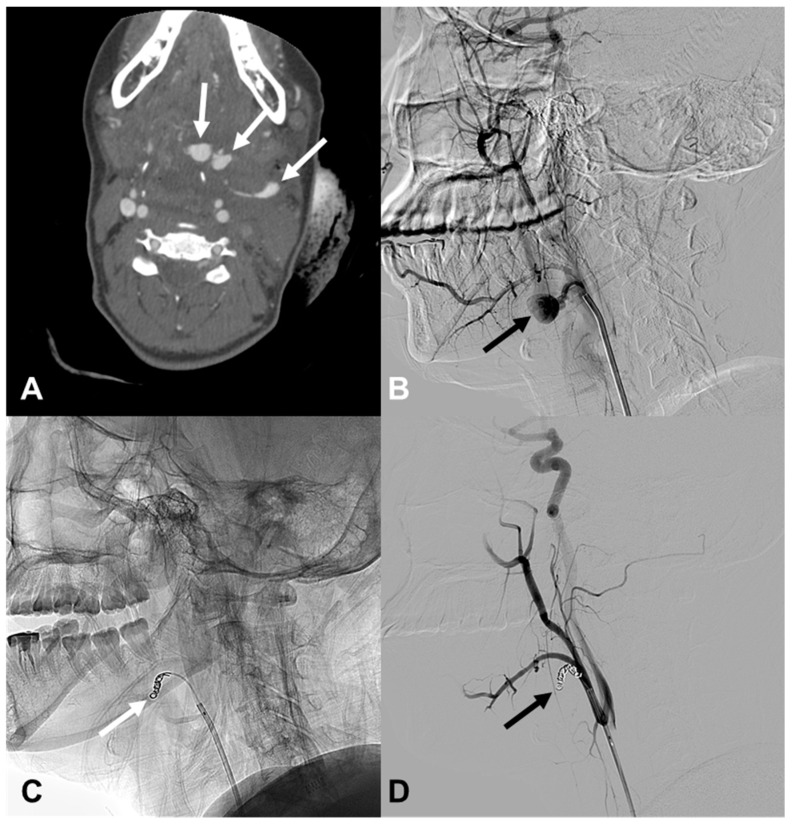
A 22-year-old woman (patient 14) with a stab injury in the left lingual artery. (A) Axial CT shows contrast extravasation (arrows) in the left level I and II. (B) Left external carotid angiography shows a large pseudoaneurysm at the left lingual artery (arrow). (C,D) The pseudoaneurysm is no longer opacified after coil embolization with three micro coils (Tornado, 6/2, 5/2, 3/2) (arrows).
